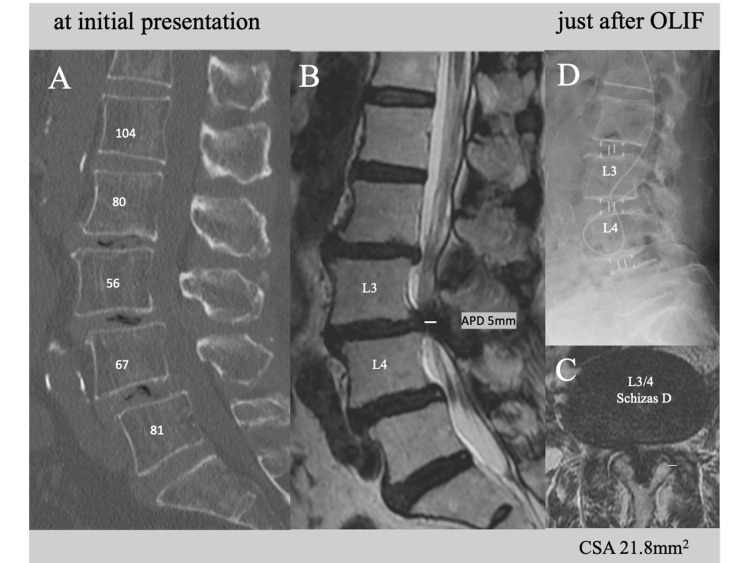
Preoperative CT and MRI images and lateral lumbar X-ray immediately after OLIF of Case 3 MRI and CT images of an 80-year-old woman (Case 3) showing (A, B, C) moderate to severe canal stenosis (Schizas B at L2/3 and L4/5 and Schizas D at L3/4), as well as degenerative spondylolisthesis at L3 and L4, and (B) very low CT-Hounsfield unit values at L2–L4. A lateral lumbar X-ray (D) taken immediately after OLIF showing significant restoration of the posterior disc height and spondylolysis without endplate injury at any level. OLIF: oblique lumbar interbody fusion, APD: anteroposterior diameter, CSA: cross-sectional spinal canal area
